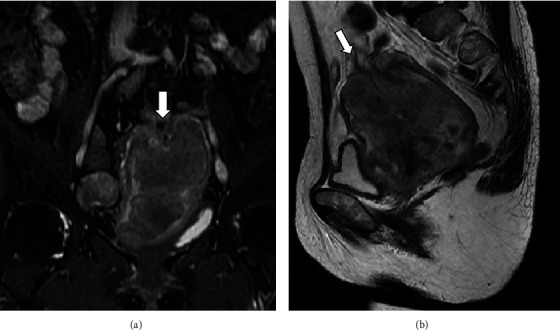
T2-weighted (a) coronal and (b) sagittal MRI images taken before surgery. The arrow shows the inverted fundus of the uterus. The uterus is U-shaped.
